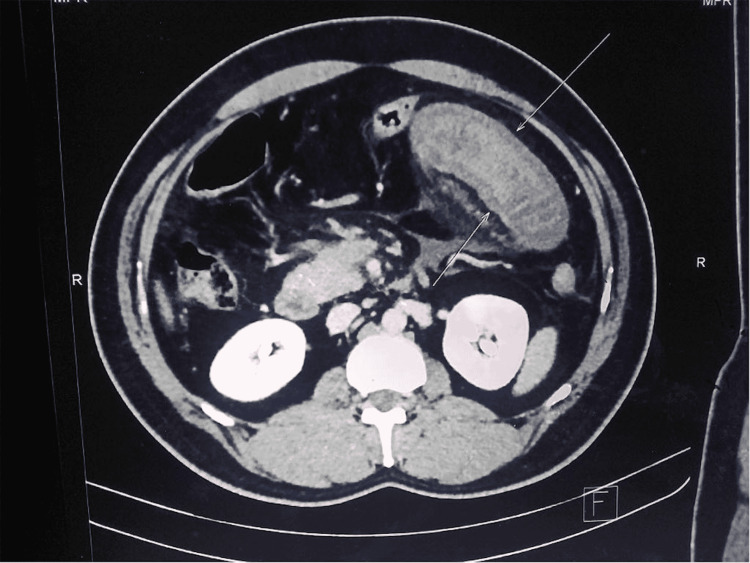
CT axial cross-section showing dilated small bowel loop.
